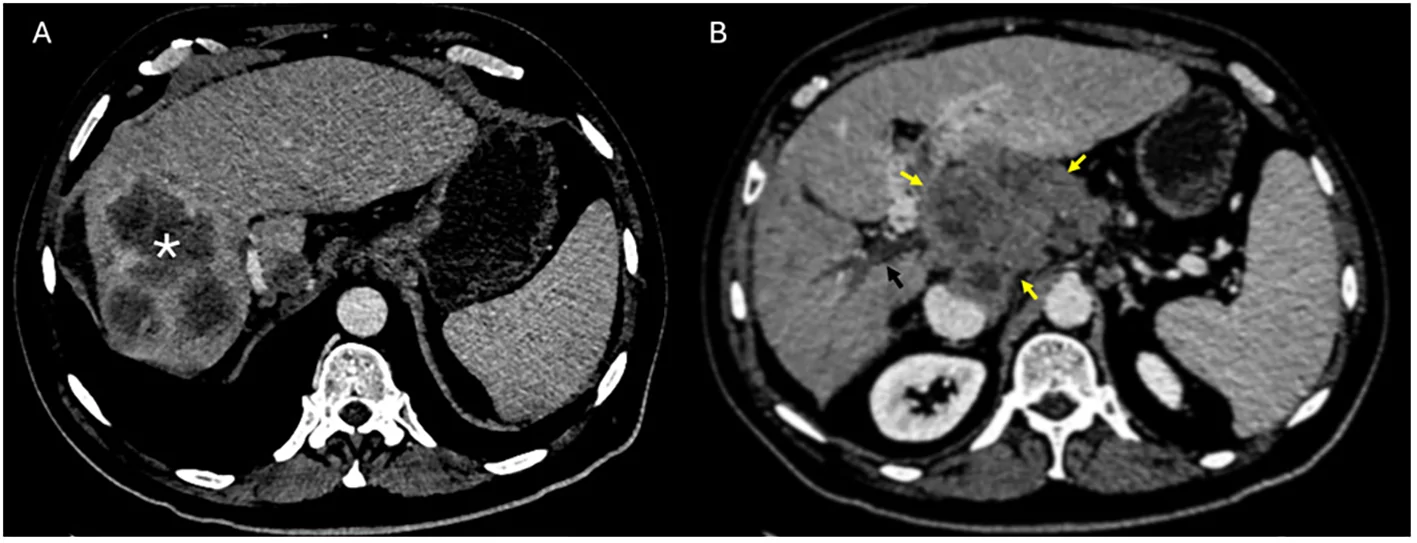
Follow-up CT scan (May 2025). (A) Tumor progression with increased size of hepatic lesions (white asterisk). (B) Necrotic hilar lymphadenopathy (yellow arrows) and extension of portal vein tumor thrombosis (black arrow).
